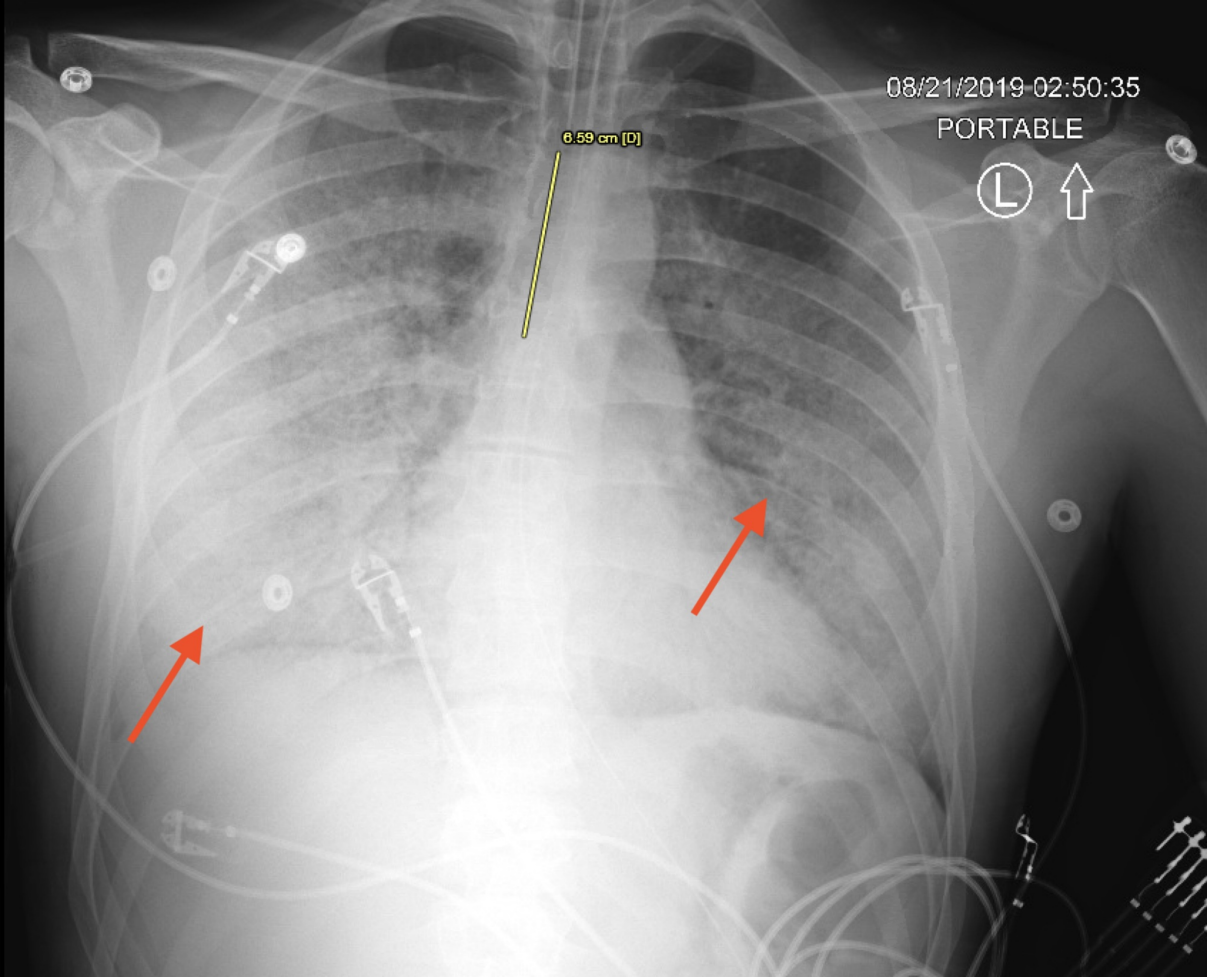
Chest x-ray (CXR) on Day 2 of admission post-intubation (yellow line showing the level of the endotracheal tube). Rapidly worsening ground-glass opacities are seen throughout the lungs with relative subpleural sparing (red arrows).
